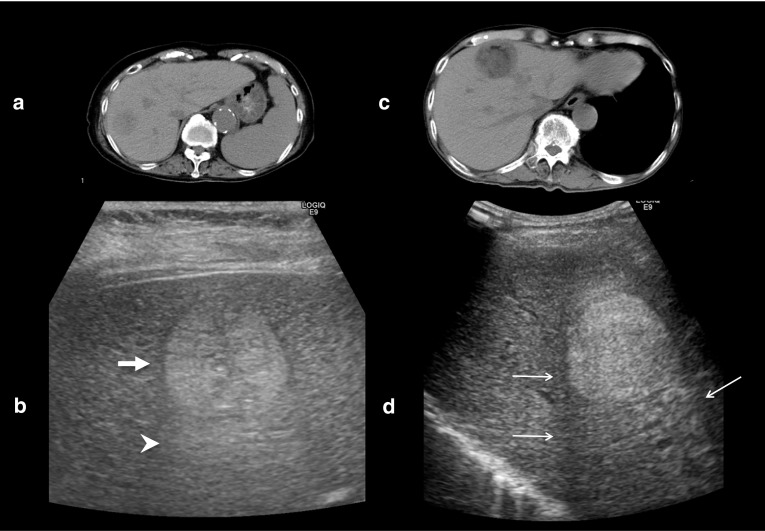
A case of newly developed hepatocellular carcinoma (HCC) (maximum diameter 26 mm) in Segment 7. Computed tomography (CT) shows a low attenuation area in Segment 7 (a). Conventional ultrasound shows a mosaic pattern nodule with posterior wall enhancement (arrowhead) and halo image (thick arrow) (b). A case of hepatitis C virus-related cirrhosis and a newly developed HCC in Segment 4 (c). Conventional ultrasound shows a high echo nodule with a typical lateral shadow (thin arrows) (d)
